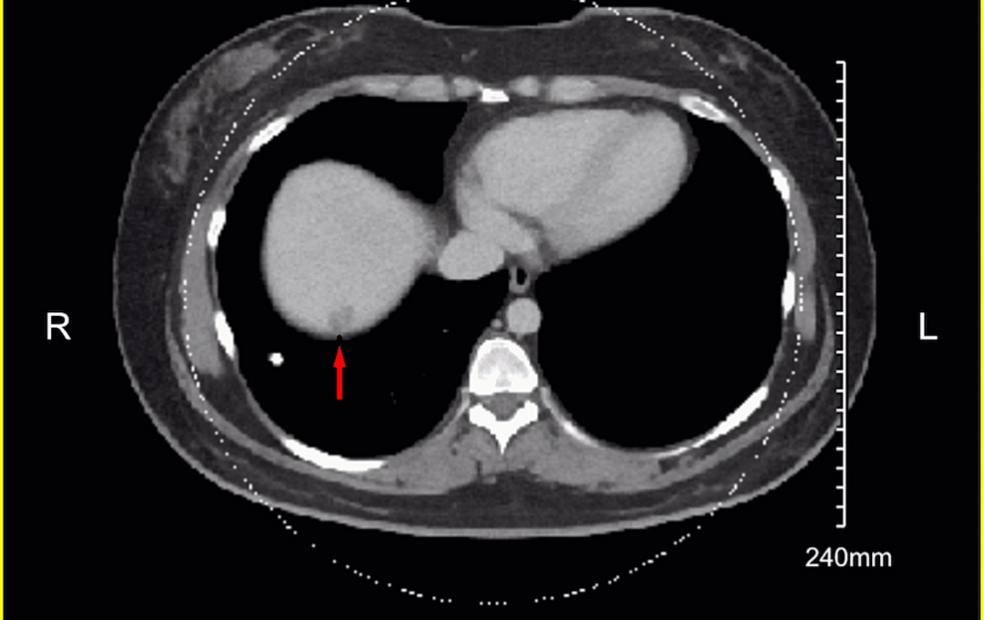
CT right hepatic dome of the liver Hypoattenuating focus in the right hepatic dome of the liver (red arrow)
